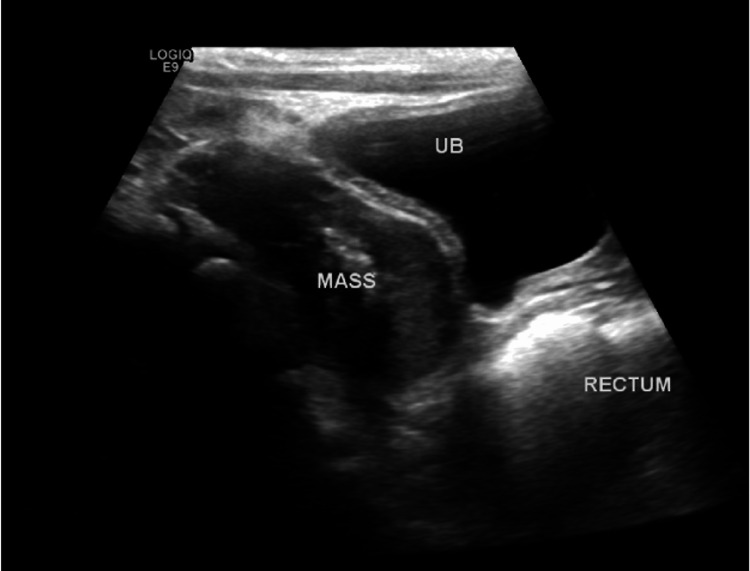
Greyscale ultrasound image demonstrating a large soft tissue mass (labeled) in the pelvis with internal vascularity and scattered calcifications.
